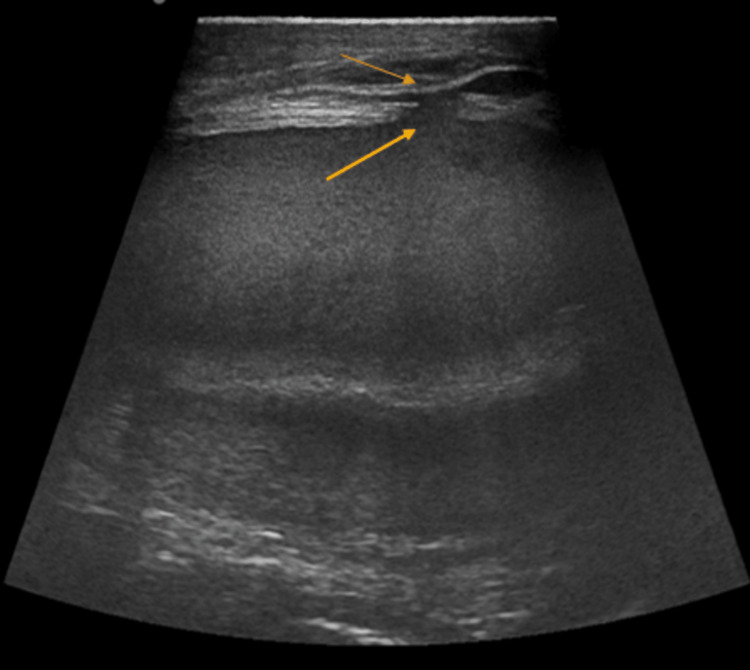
Ultrasound image of the left scrotal sac using linear probe showing hypoechoic collection with debris giving falling snow appearance with disruption in the tunica layers (yellow arrow), and intact tunica vaginalis layer (orange arrow).
